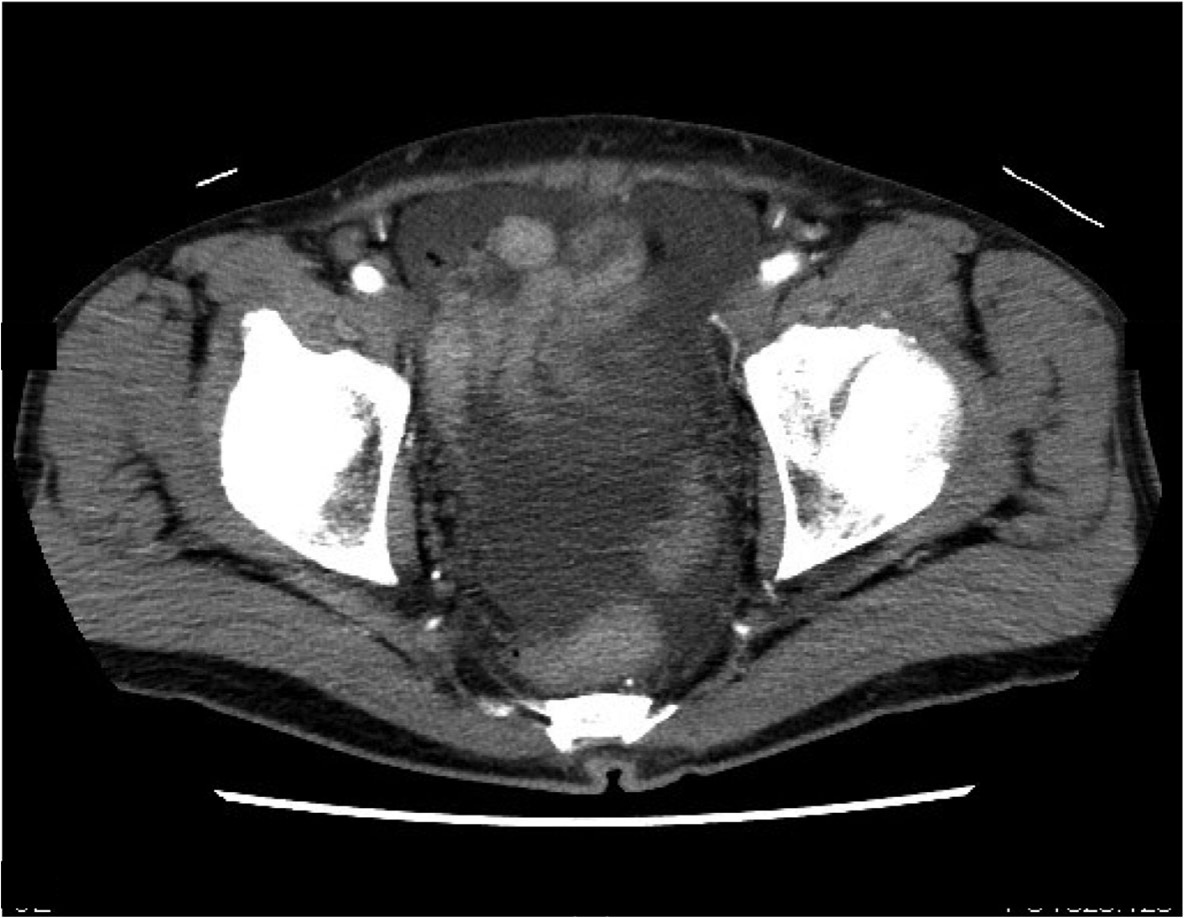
Contrast-enhanced computed tomography image before staging laparoscopy showing pelvic ascites
